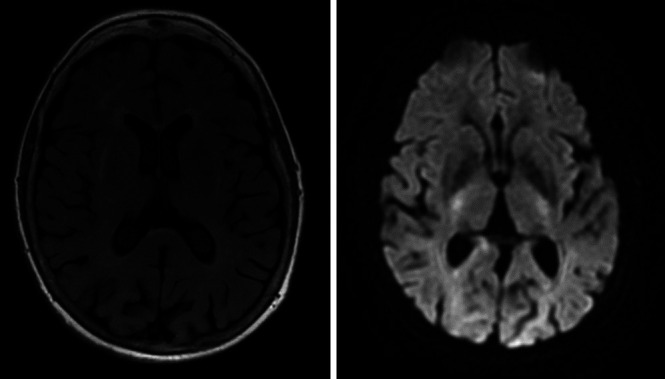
Axial brain MRI images showing multiple white matter hyperintensities; left: fluid-attenuated inversion recovery brain MRI sequence; right: diffusion-weighted imaging brain MRI sequence.
